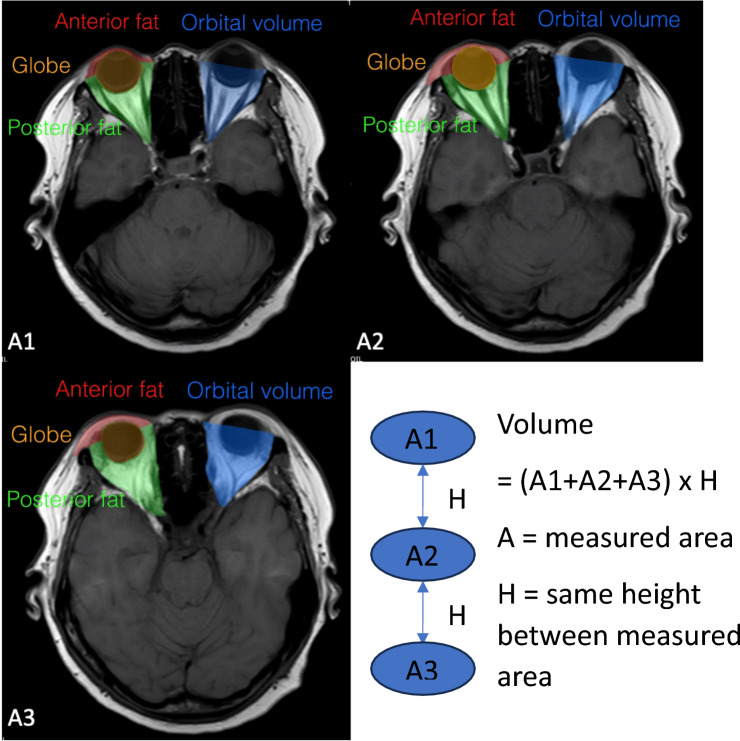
Measurement from axial view of MRI and formula for volume calculation
